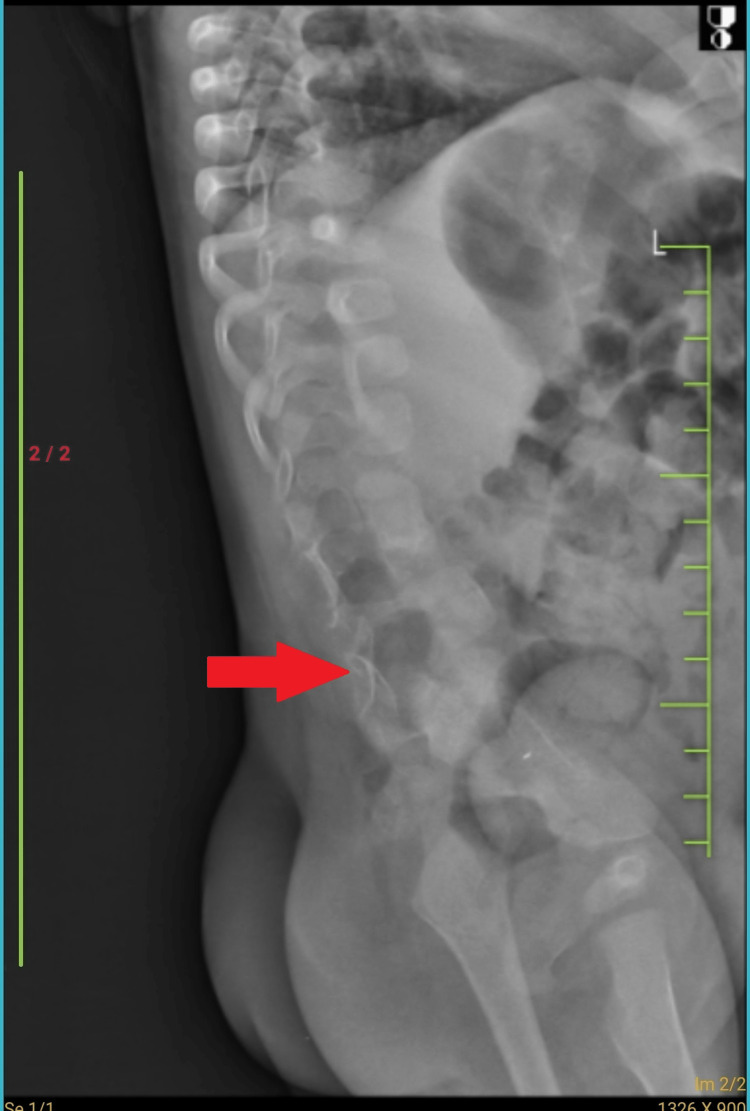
X-Ray lumbosacral spine lateral view showing altered intervertebral discs showing concavities along the adjacent end plates.
